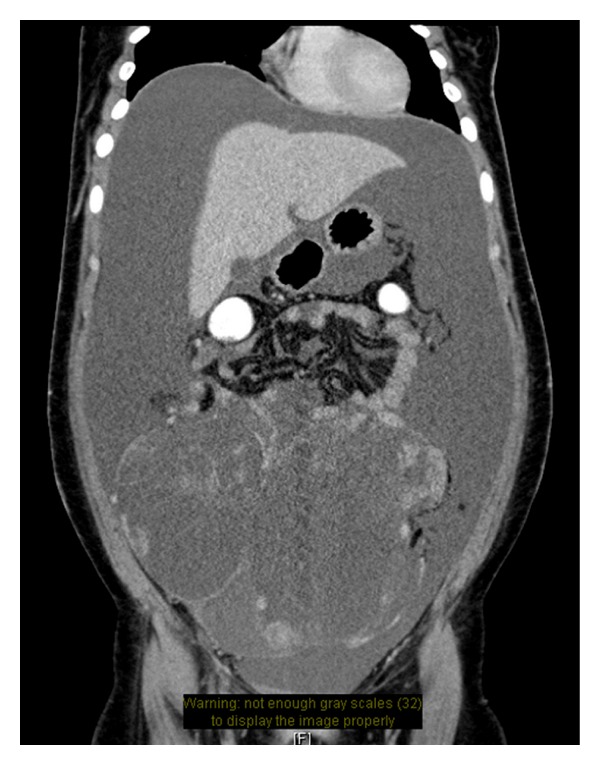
A chest, abdominal, and pelvic contrast-enhanced computed tomography (CT) scan at the portal venous phase with rectal contrast showed a huge, intraperitoneal, complex, cystic, and multilocular lesion, extending from pelvis up to midabdomen just above umbilicus, collectively measuring 13.5 × 23.3 × 21.5 cm. The source of lesion was more likely to be left ovary which was probably ruptured and leaked into the above-mentioned cystic lesion. In addition, large ascites was identified. No significant lymphadenopathies in abdomen and pelvis were noticed.
